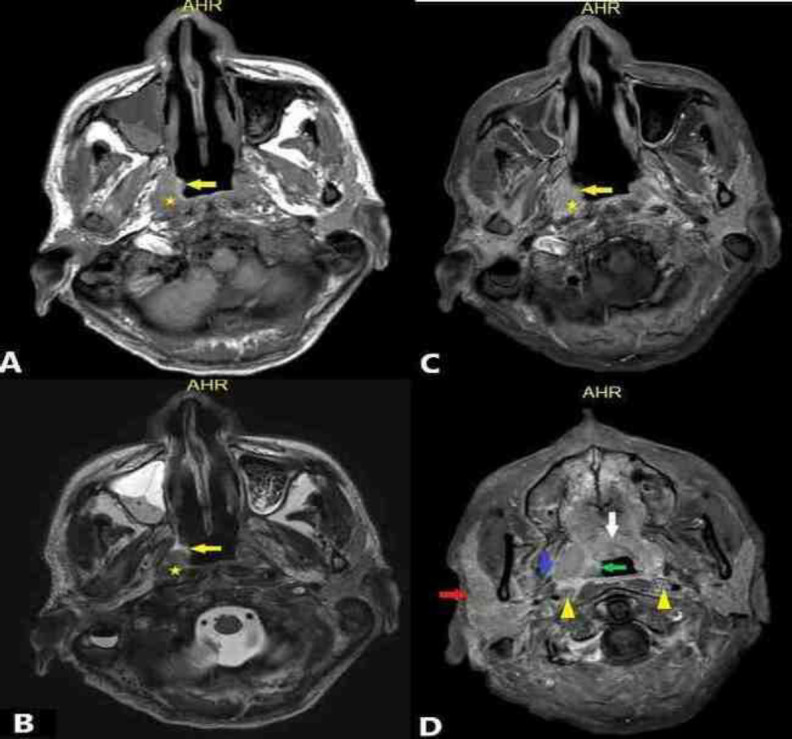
MRI neck in axial T1-weighted (A), T2-weighted (B) and post-contrast (C and D). Figure A, B and C show right nasopharyngeal lesion (yellow arrow) and obliteration of right FOR (star). Figure D shows the extension of the lesion to the right oropharynx (green arrow), right parapharyngeal (blue arrow), soft palate (white arrow) and right parotid (red arrow). Bilateral retropharyngeal LN enlargement is also seen in Figure D (yellow arrowhead)
